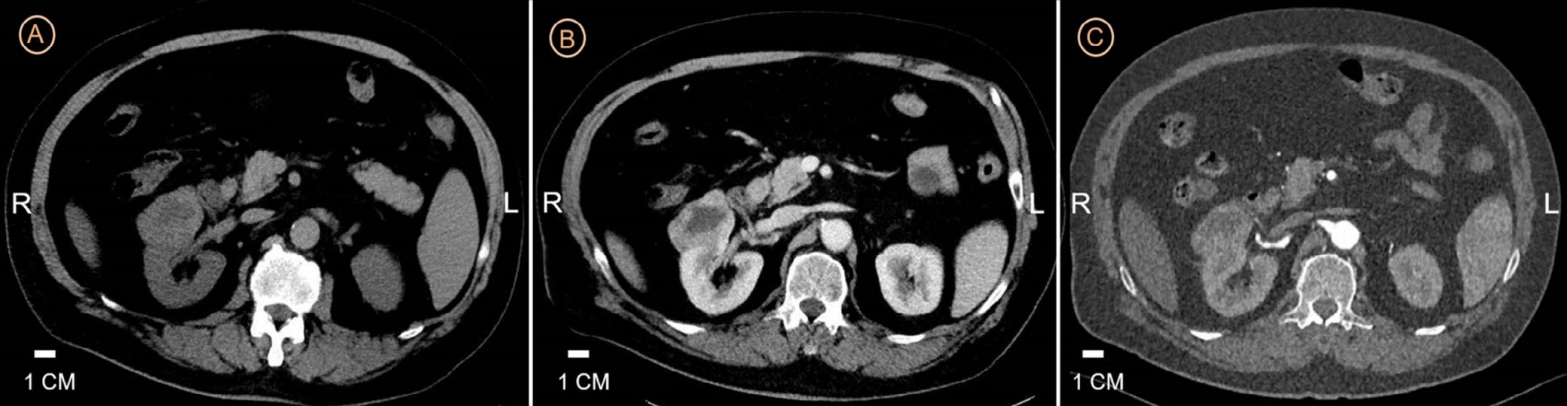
Axial CT images of the patient before radical nephrectomy, including (A) (plain axial CT), (B) (contrast‐enhanced axial CT in the arterial phase), and (C) (axial CT angiography, CTA).
